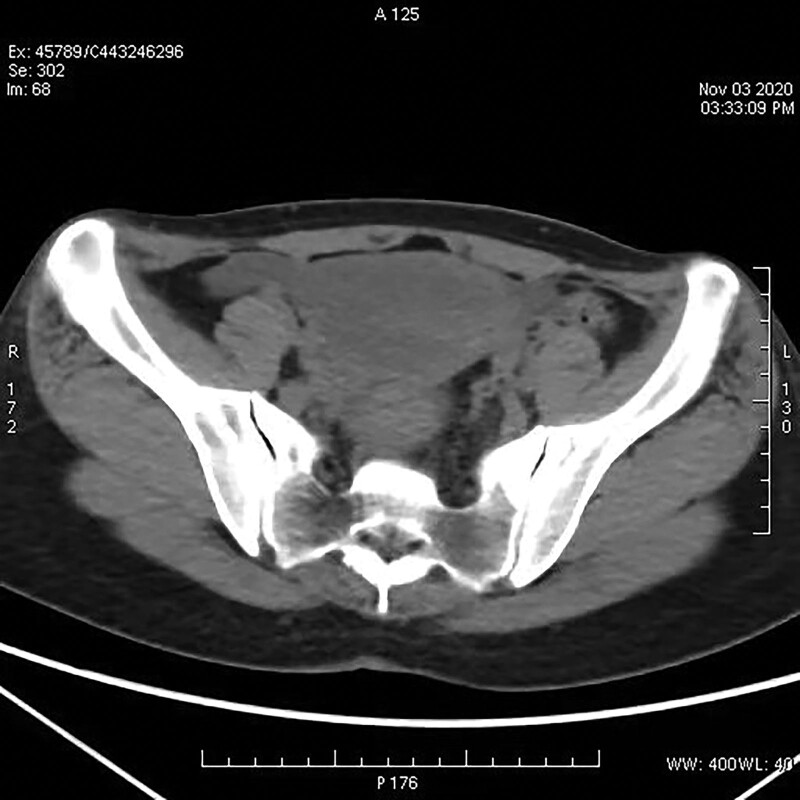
CT scan of the abdomen shows a pelvic mass with a malignant tendency of mass, smaller than before.
